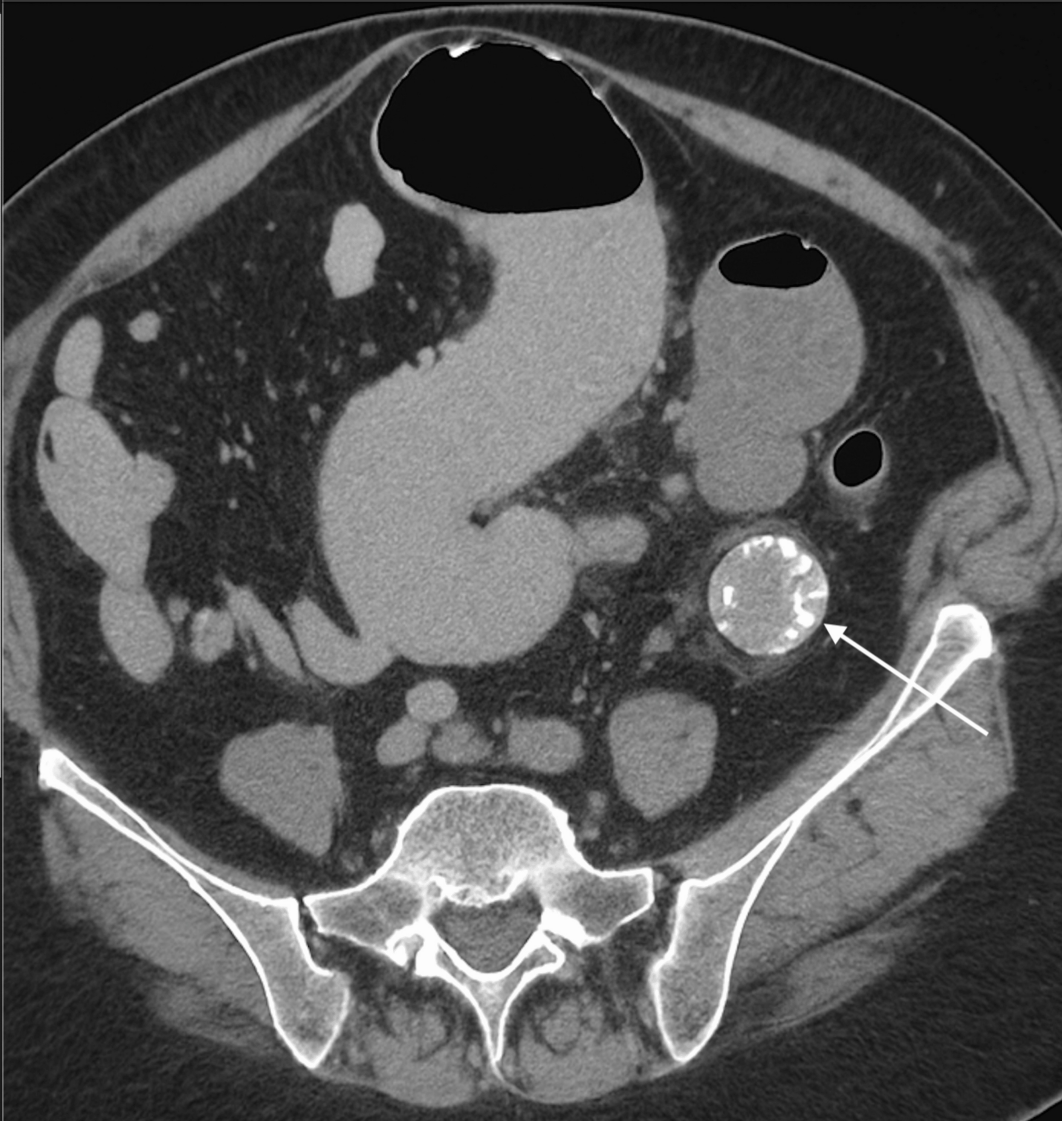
CT axial view showing ectopic gallstone (arrow). Axial CT image showing a hyperdense ectopic gallstone (white arrow) located in the distal jejunum/proximal ileum. Pneumobilia is also visible in the intrahepatic biliary tree. These findings support the diagnosis of gallstone ileus. Scale bar = 1 cm.
